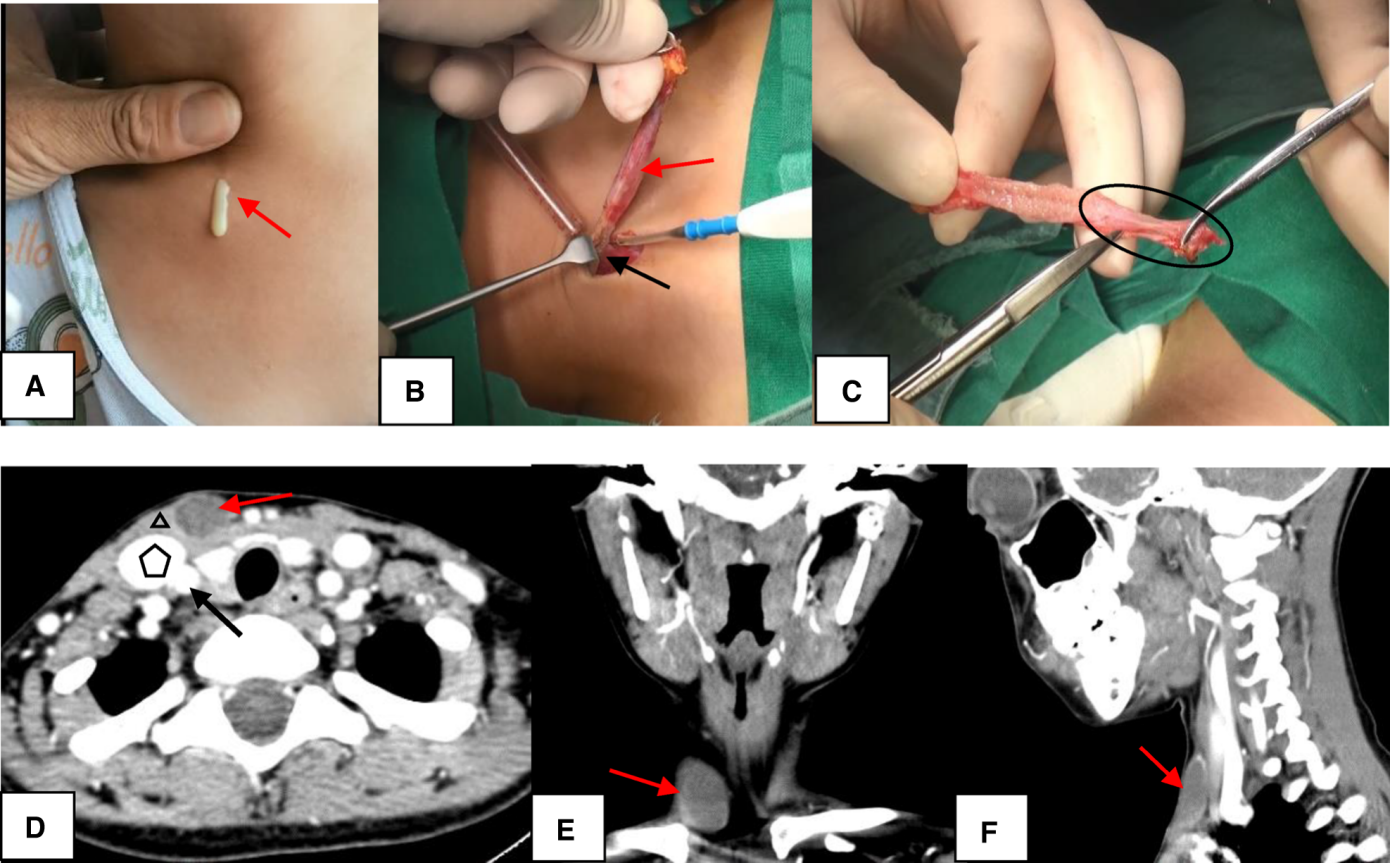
The Bailey type I. (A) The sinus was presented as having a skin pit on the lateral neck and discharge from the skin opening (red arrow). (B) The sinus (red arrow) terminated at the surface of SCM (black arrow). (C) The end of the sinus closed to form a muscle bundle (circle) ending at the surface of SCM. (D–F) On CT, the lesion (red arrow) lied along anterior surface of SCM (triangle) just deep to platysma, not in contact with the carotid sheath (pentagon: internal jugular vein; black arrow: arteria carotis communis). D, axial position; E, coronal position; F, sagittal position; SCM, sternocleidomastoid muscle; CT, computed tomography.
